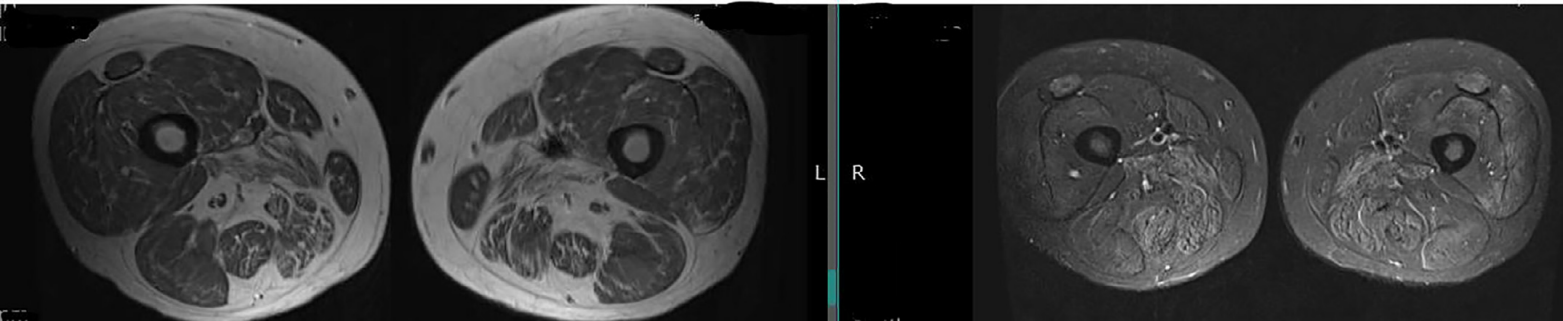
Magnetic resonance imaging of the bilateral thighs showing fatty replacement on T1 sequence (left) and moderate edema on STIR (fat suppressed) sequence (right), consistent with myositis.
